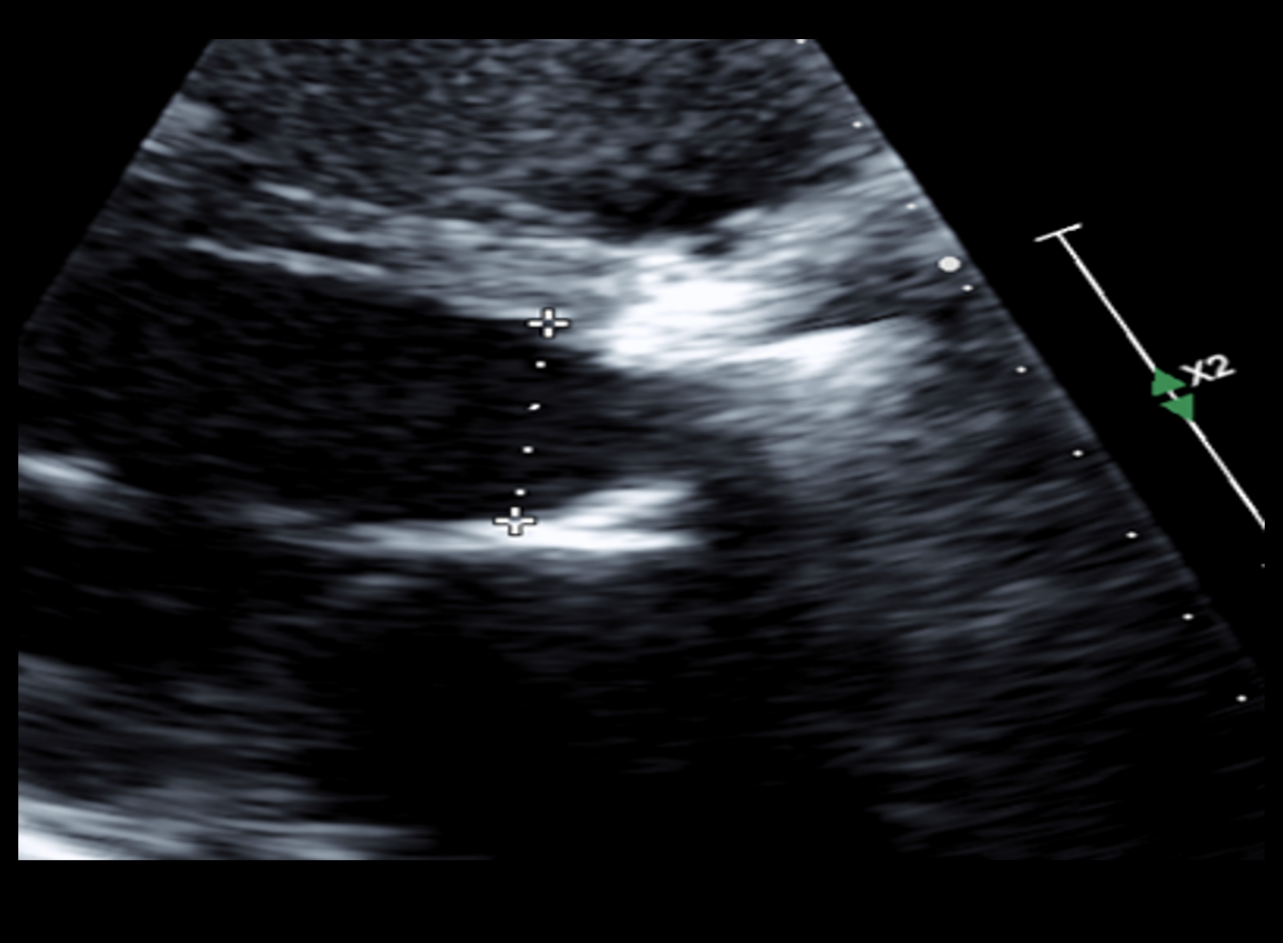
Echocardiogram showing Ball and Cage, and Starr Edwards valve
